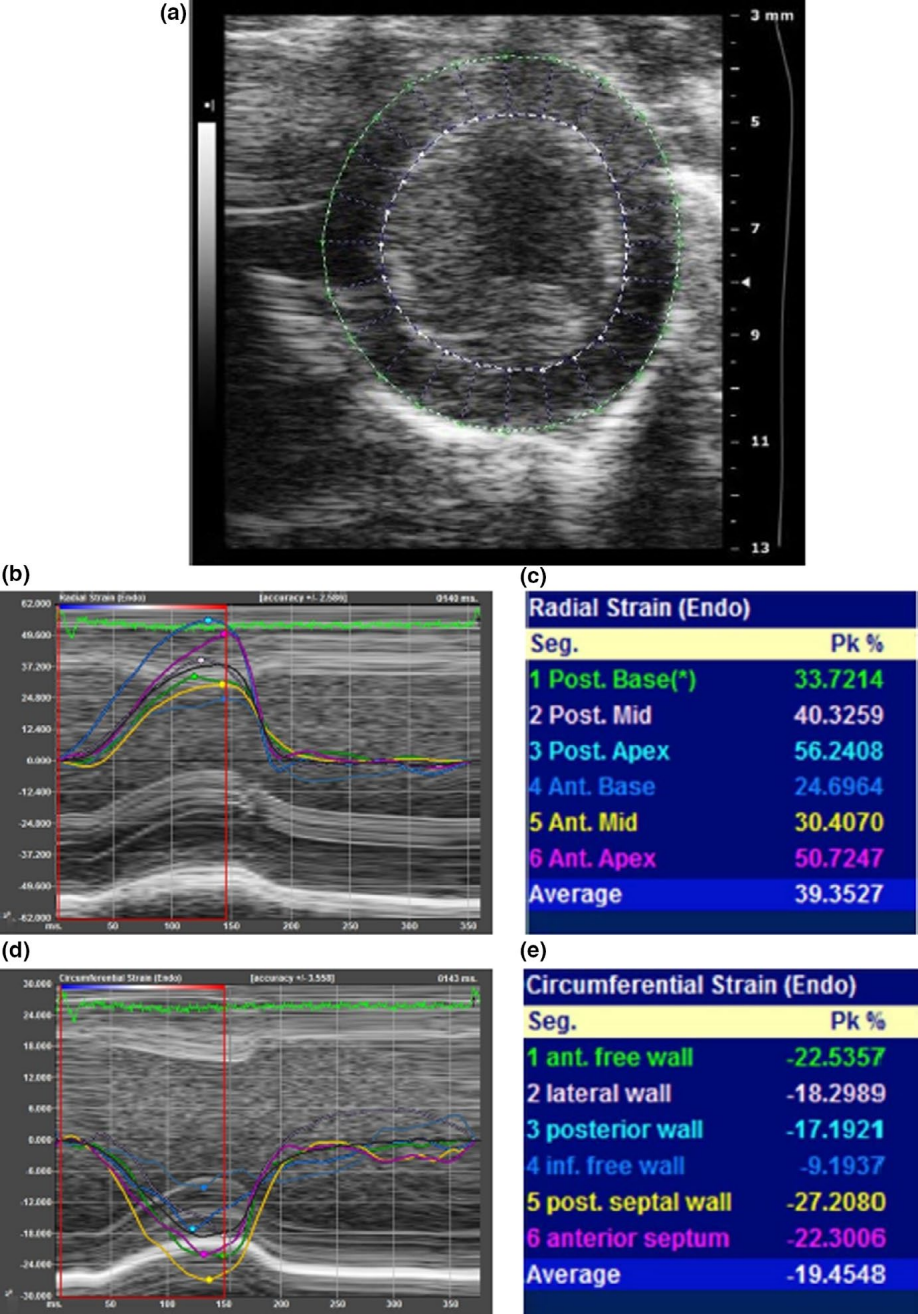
Myocardial strain analysis in hamsters from parasternal short‐axis view (PSSAX). (a) Analysis of endocardial (white dashed line) and epicardial (green outline) tracings; (b) radial strain curves; (c) radial strain peak systolic values; (d) circumferential strain curves; (e) circumferential strain peak systolic values and segmental nomenclature. Endocardial strain values are represented for global (white line = average of segments), anterior (1); lateral (2); posterior (3); inferior free (4); posterior septal (5); and anterior septal (6) segments
